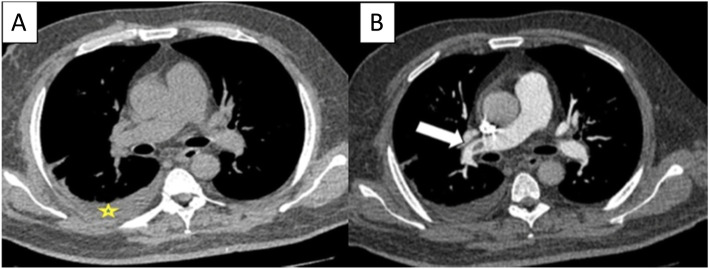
a Axial non contrast CT image. b Axial CTPA image shows a case of false-negative finding of pulmonary embolism in non-contrast CT chest associated with right pleural effusion (asterisk in a), with positive finding in CTPA as hypo dense filling defect seen partially occluding right main pulmonary artery (arrows in b)
